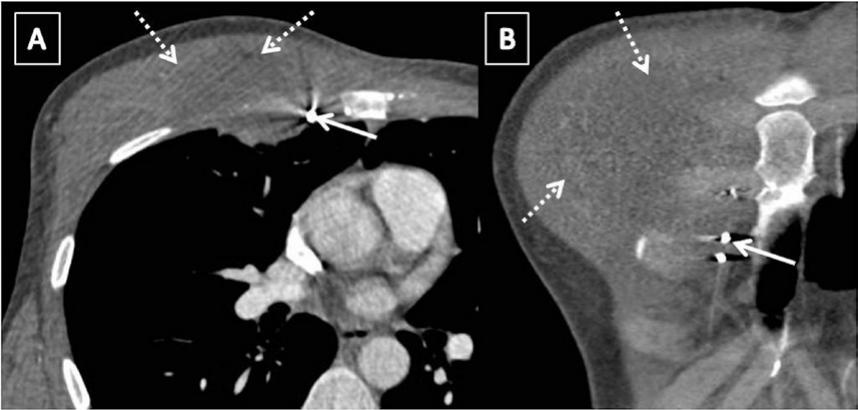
Post-coiling CT: CT images (A and B) following coil embolization show no residual filling of the sac with coil mass in situ (arrow) and resolving residual hematoma (dashed arrows).
